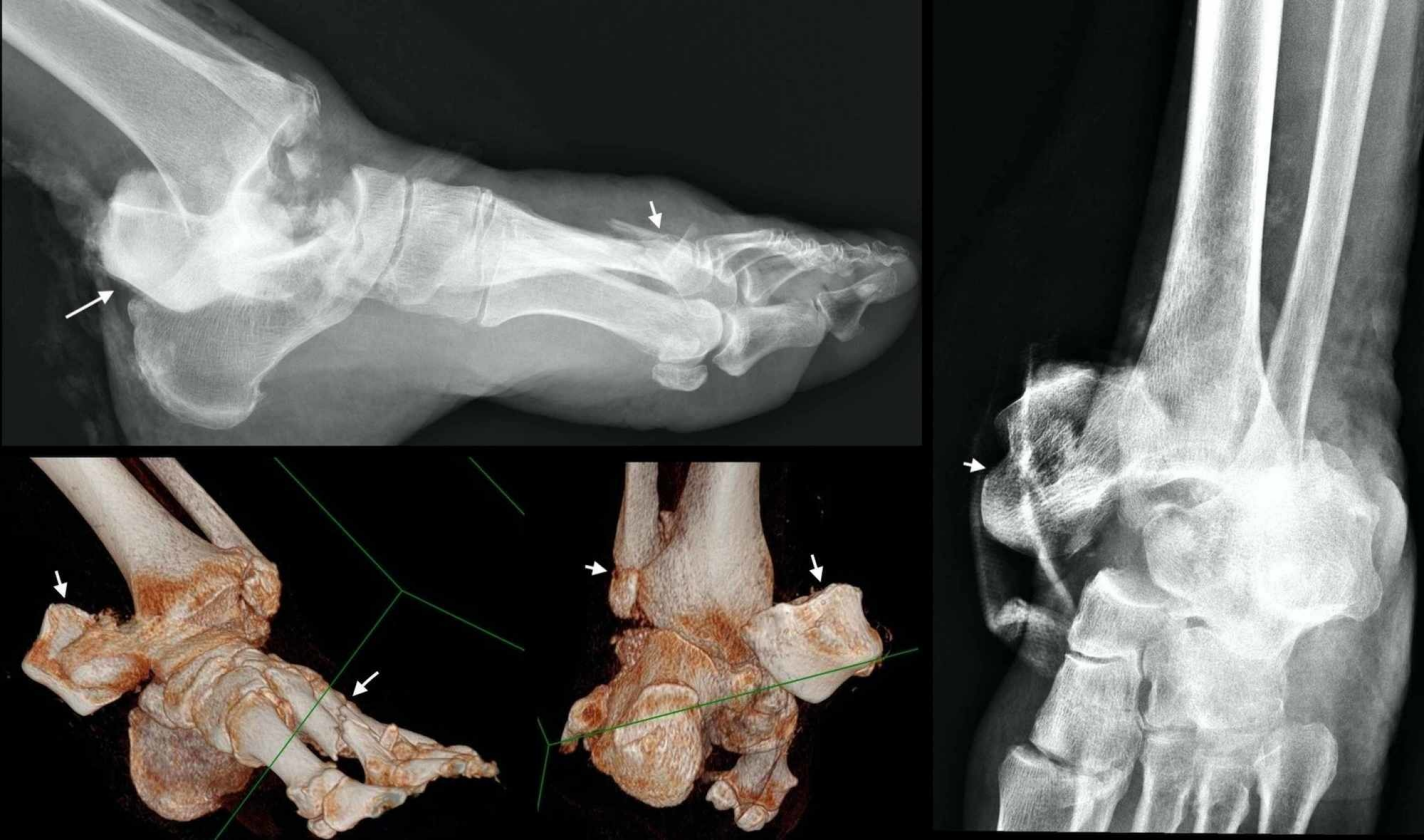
Radiographs and a CT scan of the left ankle showing an extrusion of the talus with multiple fractures (distal fibula, tarsal and metatarsal bones)
